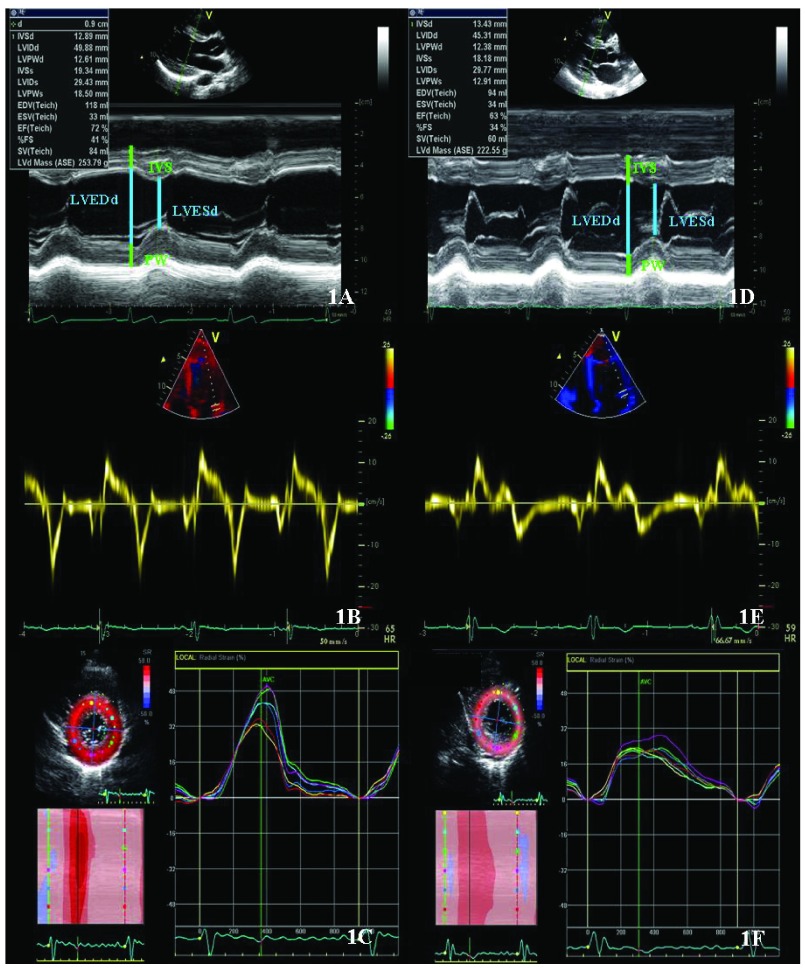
Echocardiographic assessment of an athlete with left ventricular hypertrophic adaptation (Figure 1: A, B, C) and a patient with a mild form of hypertrophic cardiomyopathy (Figure 1: D, E, F). Patients with hypertrophic cardiomyopathy present smaller LV end-diastolic diameters, reduced radial strain values and reduced velocities of the mitral annulus as compared to athletes.
